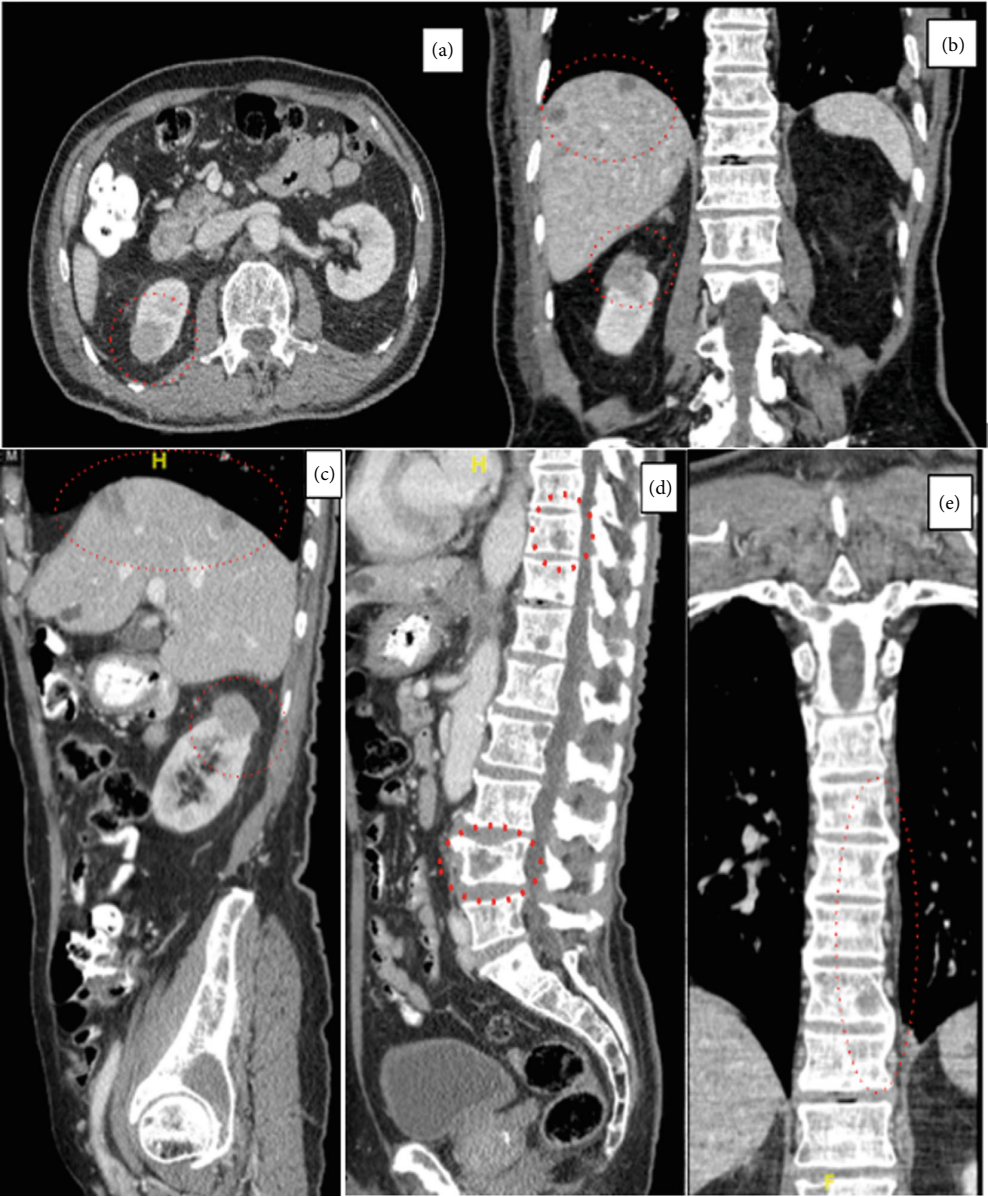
Computed tomography imaging demonstrating widespread metastatic disease. (a) Axial CT abdomen showing right renal metastasis. (b) Coronal CT abdomen revealing multiple liver and right adrenal metastases. (c) Sagittal CT abdomen with combined renal and hepatic involvement. (d) Sagittal CT spine showing extensive lumbar vertebral metastases. (e) Coronal CT spine with multiple skeletal metastases.
